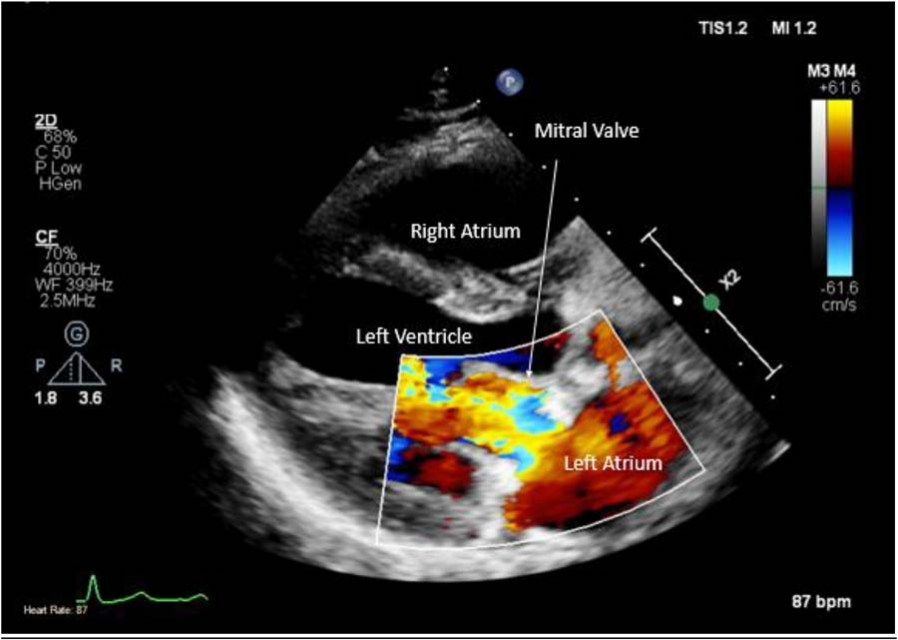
Post-operative TTE with Doppler depicting mitral valve repair.
